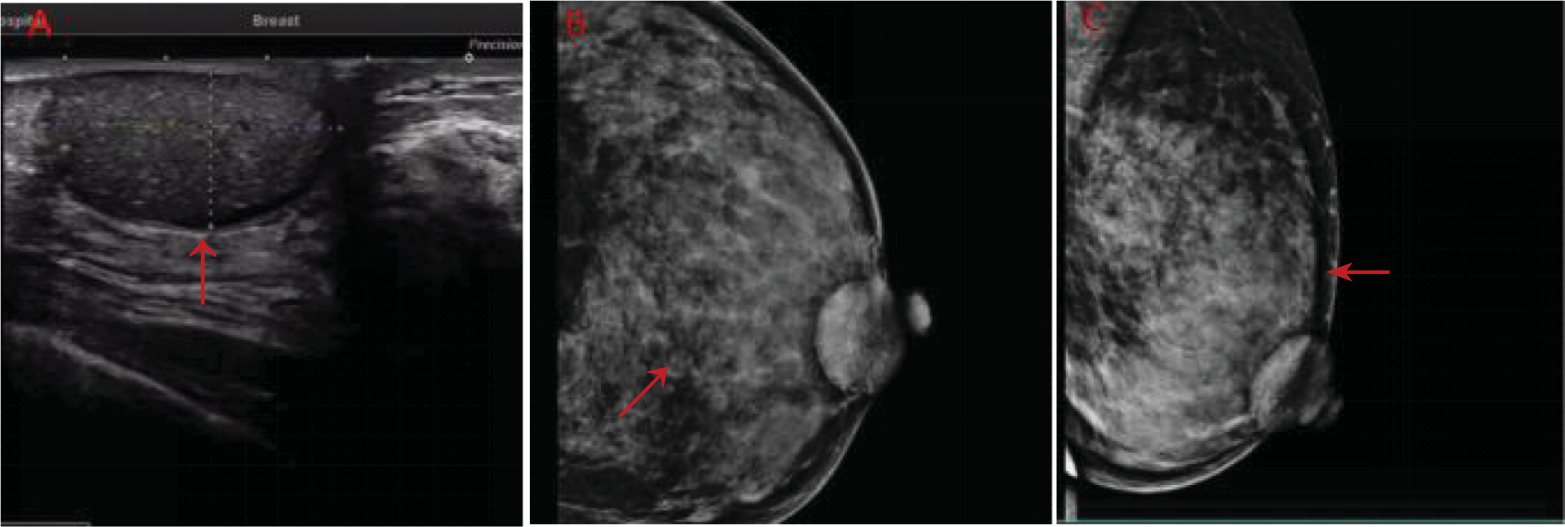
(A) Color ultrasonography evaluation of the patient before surgery. (B, C) Preoperative images using molybdenum target mammography (red arrows indicate lesions).
